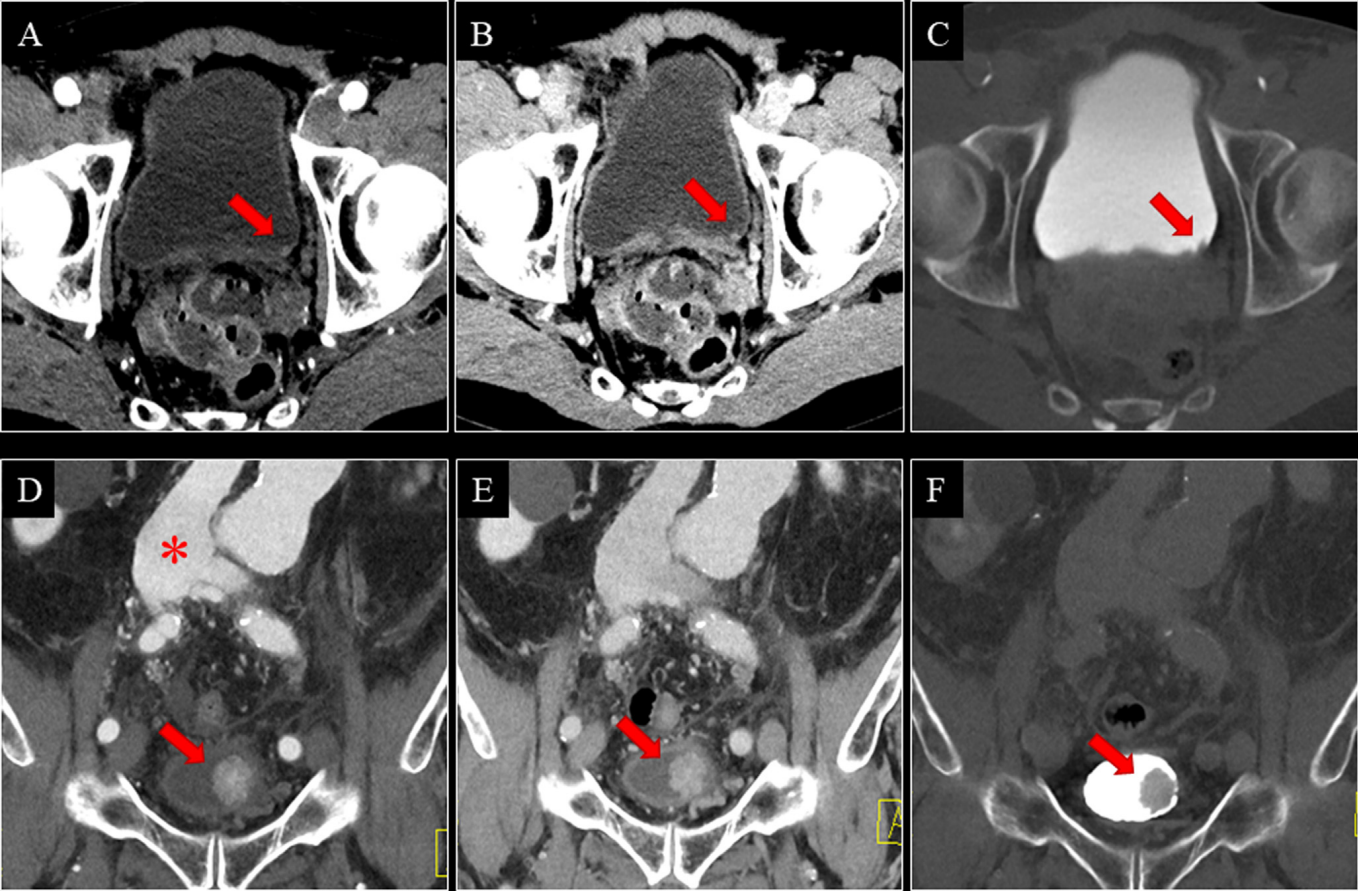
(A–C) A 73-yr-old male with a pTa tumour in the bladder. The experimental CT was negative for bladder UC in both reading teams. Retrospectively, the tumour (red arrow) was visible on all phases (axial CMP [A], NP [B], and EP [C]). The control CT was positive for bladder UC in both reading teams. (D–F) An 83-yr-old male with a solid tumour in the bladder. Both the control and the experimental CT were positive for bladder UC in both reading teams. The tumour (red arrow) was visible on all phases (coronal CMP [D], NP [E], and EP [F]). An ilioiliac fistula caused arterial filling of the inferior vena cava (red asterisk). Large vesical varices due to the ilioiliac fistula made the patient unfit for TURB, and he received radiotherapy. CMP = corticomedullary phase; CT = computed tomography; EP = excretory phase; NP = nephrographic phase; pT = pathological tumour stage; TURB = transurethral resection of the bladder; UC = urothelial carcinoma.
